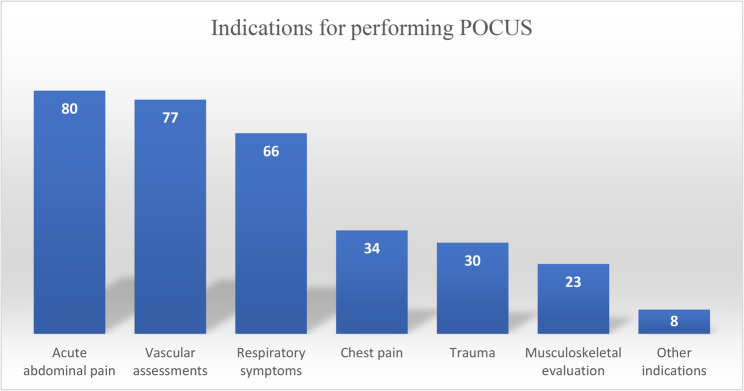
Clinical indications for point-of-care ultrasound among current users (*n* = 101). Values represent number of respondents
